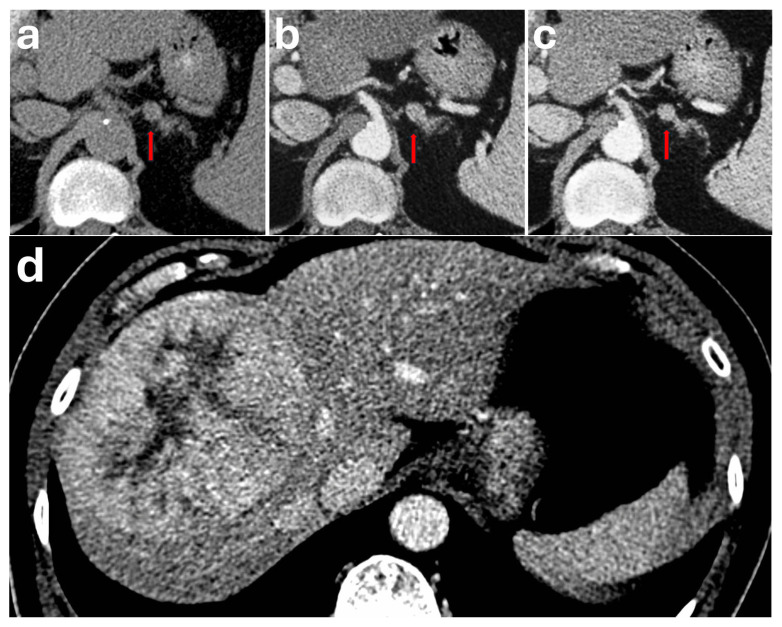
Patient with a prior surgical history of hepatic VII segmentectomy for hepatocellular carcinoma (HCC) presented with rising alpha-fetoprotein levels. CT demonstrated a 1.4 cm homogeneously enhancing nodule arising from the body of the left adrenal gland (red arrows). It demonstrated 44 HU on the pre-contrast phase (a), 105 HU on the portal venous phase (b), and 58 HU on the delayed phase (c). Absolute washout was 77.0% and relative washout was 44.8%. Biochemical markers were normal. Subsequent resection of the left adrenal gland confirmed metastasis from HCC. (d) Initial CT scan demonstrating the primary hepatocellular carcinoma localised to segment VII of the liver. There was no evidence of distant metastasis to the adrenal glands on the initial scan.
